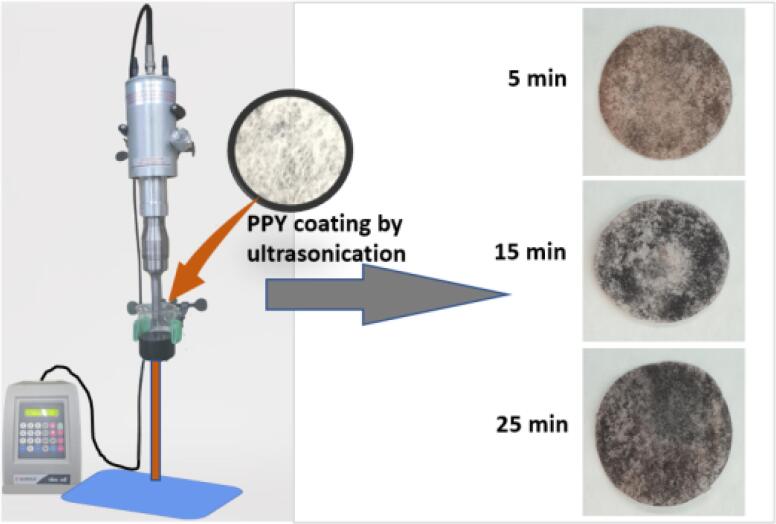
Ultrasonication-assisted coating of PPY particles on fluorinated nonwoven fabric.
